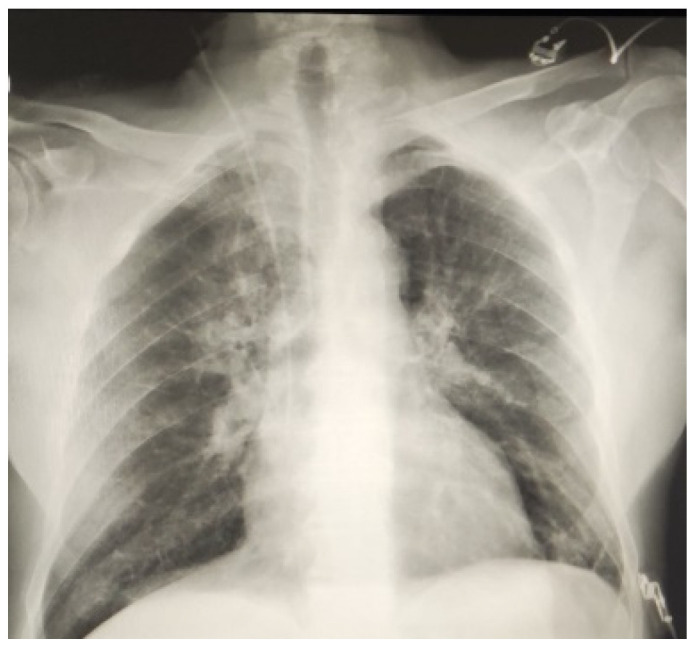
The chest X-ray on a patient during third postoperative day after single vessel RA-MIDCAB. The result appears satisfactory allowing for a faster recovery.
